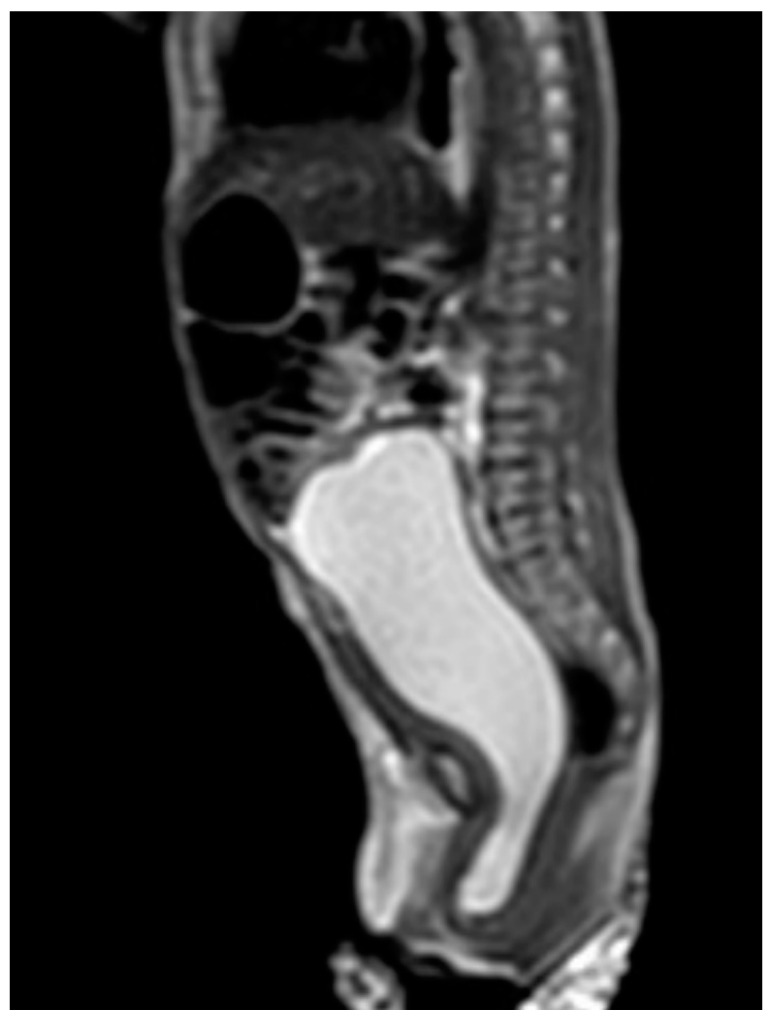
MRI of case 1: hydrometrocolpos manifested as an intraabdominal huge cystic mass.
